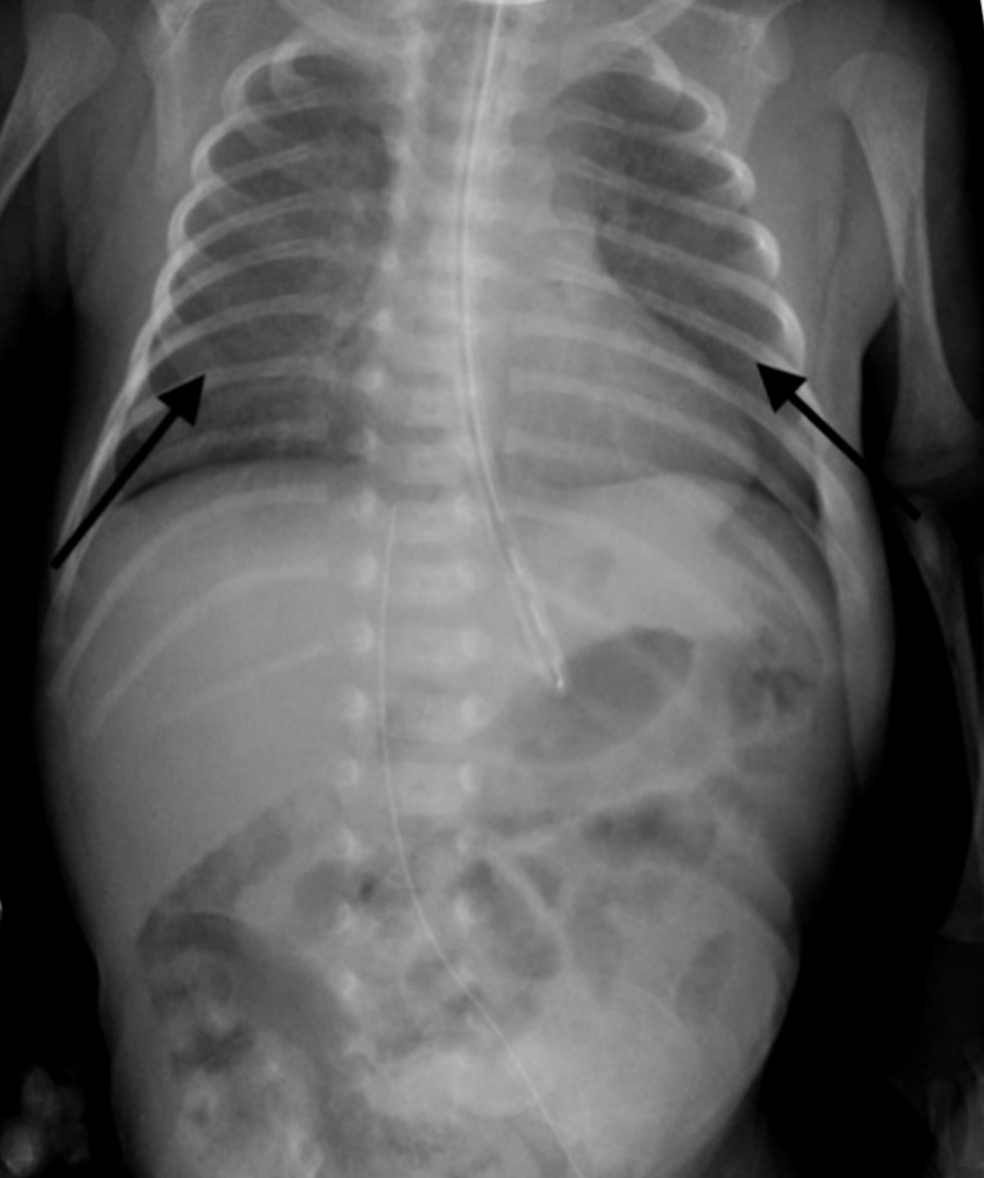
Post-extubation chest/abdomen x-ray: AP view showing significantly improved aeration of both lungs AP: anteroposterior
